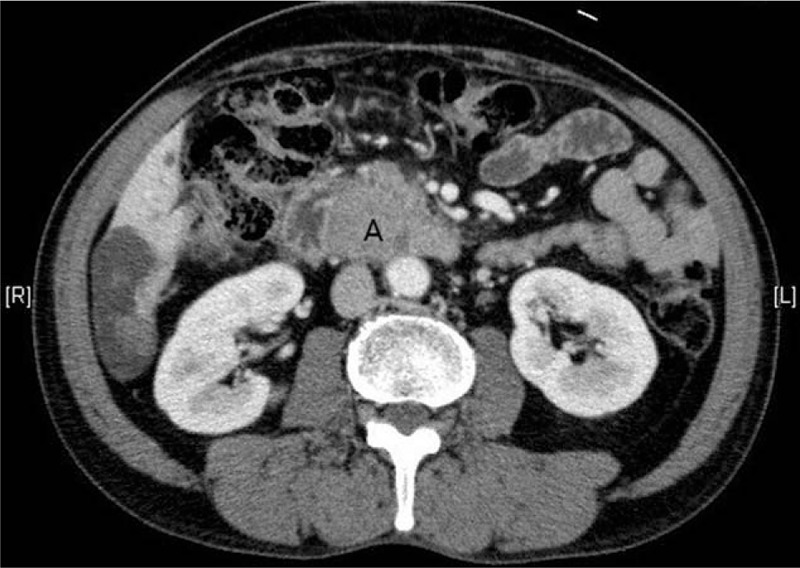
Abdominopelvic computed tomography showing a 4 × 4.5-cm-sized solid enhancing lesion in the periampullary region.
